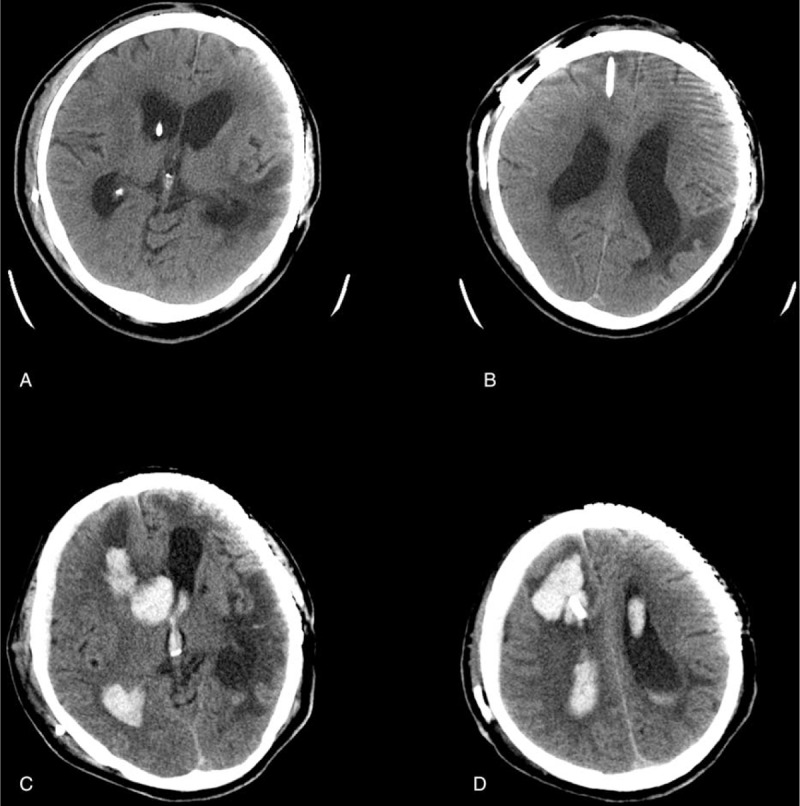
CT scans of pre- and posthemorrhage onset of the patient. (A, B) CT scan on 8th day after VP shunt showed enlarged ventricles without any bleeding. (C, D) CT scan after clinical deterioration revealed a massive intraparenchymal and intraventricular hematoma along the path of ventricular catheter. CT = computed tomographic, VP = ventriculoperitoneal.
